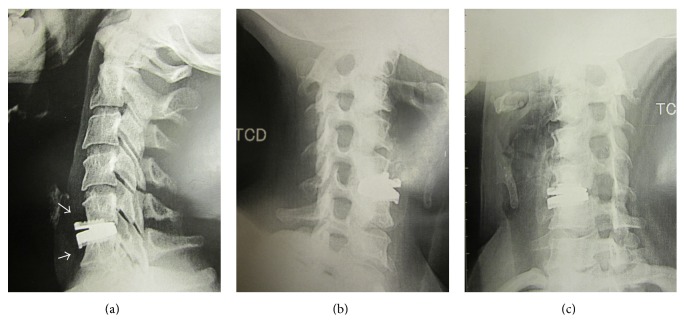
Plan radiographs performed after symptoms recurrence. The endplates adjacent to the prosthesis were shortened, making the prosthesis more protruding (a). The foramina were not narrowed (b and c).
